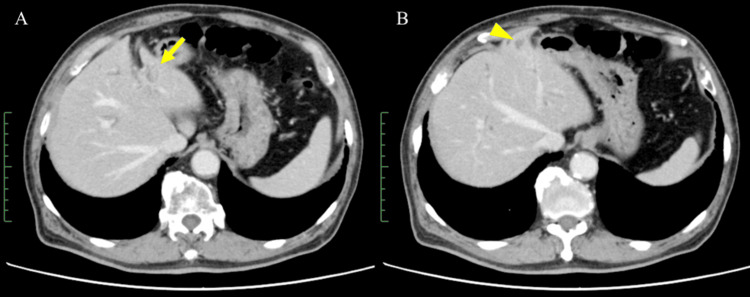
Abdominal computed tomography (CT) images at diagnosis (A, B) Abdominal CT images revealed a mass lesion in the left lobe of the liver (arrow) and dilation of the left intrahepatic bile duct (arrowhead).
